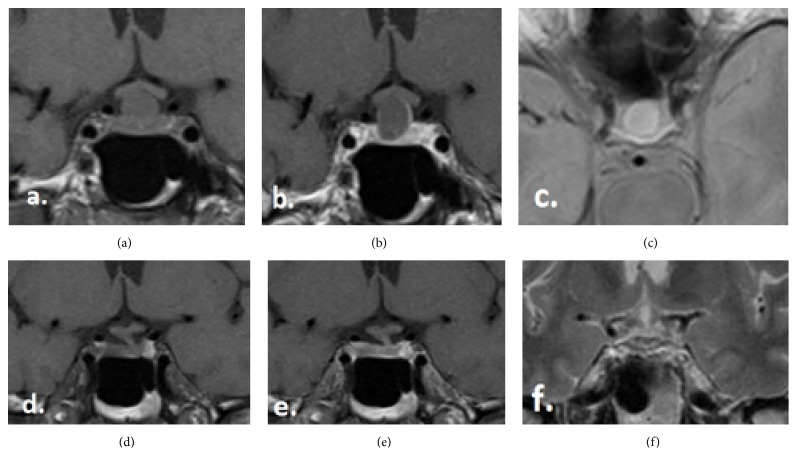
(a–c) The MRI images by the initial diagnosis, (a) T1-coronal without contrast, (b) T1-coronal with contrast, and (c) T2-axial without contrast. (d–f) The MRI images by the 1-year follow-up, (d) T1-coronal without contrast, (e) T1-coronal with contrast, and (f) T2-coronal without contrast.
